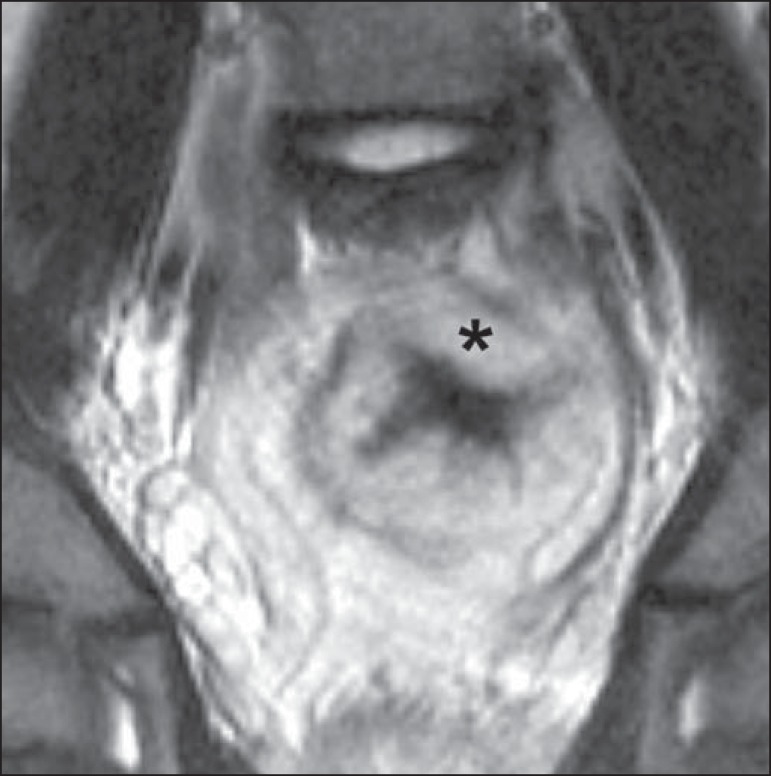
Blue rubber bleb nevus syndrome. Sagittal T2-weighted MRI scan
